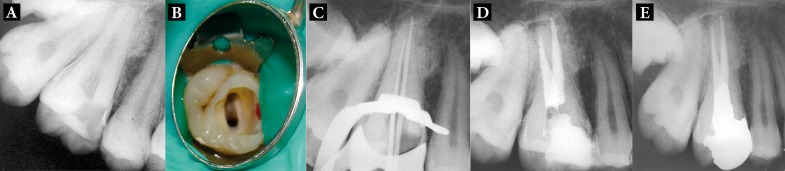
A) Preoperative radiograph of two-canalled first maxillary molar; B) Access cavity reveals one buccal and one palatal canal; C) The master apical cones confirmed the measured lengths; D) Final radiograph; E) 12 month follow-up
